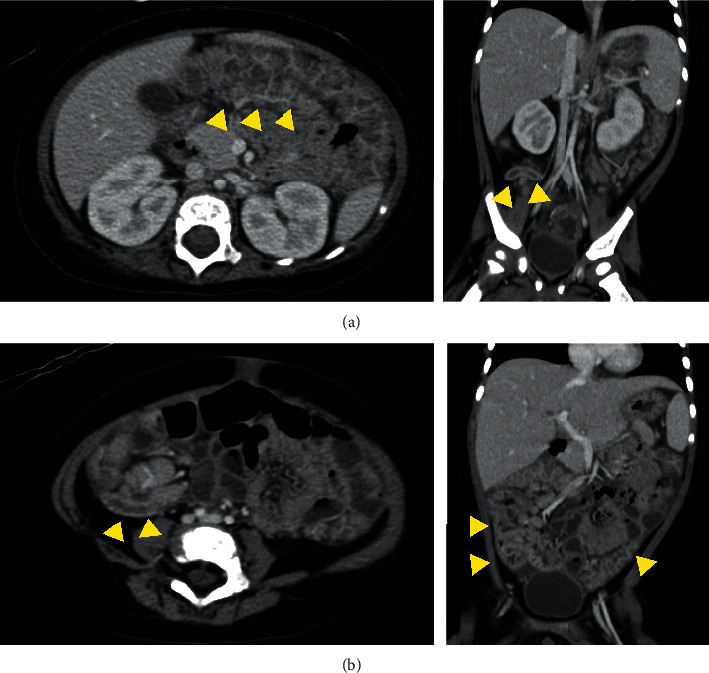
Abdominal computed tomography revealing pitting-like wall thickness of the entire colon, mesenteric lymphadenopathy of the right lower abdomen, and enlarged appendix.
